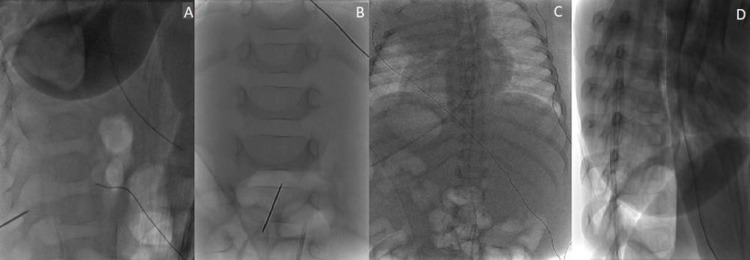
Lateral (A) and posteroanterior (B) radiographs demonstrating needle placement at the L2-3 interspace. Confirmatory posteroanterior (C) and lateral (D) radiographs demonstrate the epidural catheter extending up to approximately the T7 level.
